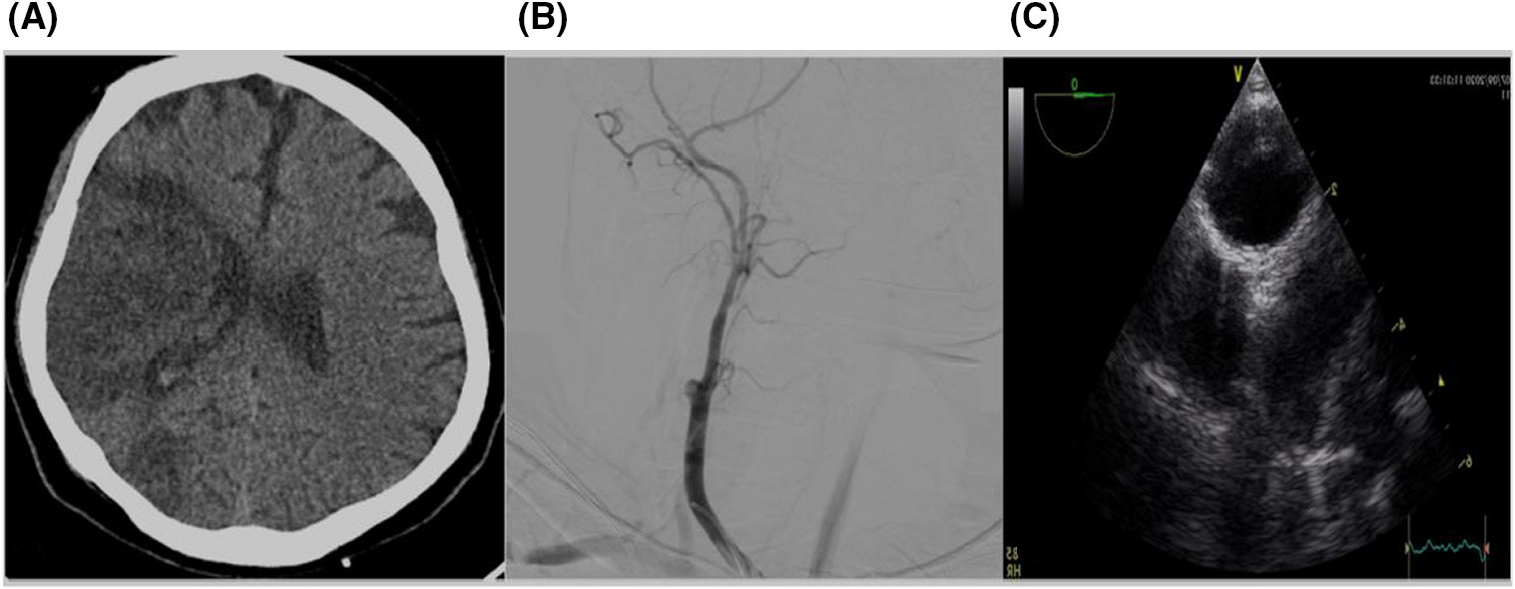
(A) CT‐scan, Hypodensity in MCA territory. (B) DSA, Rt ICA occlusion. (C) Trans thoracic echocardiography, a large aortic arch thrombus.
